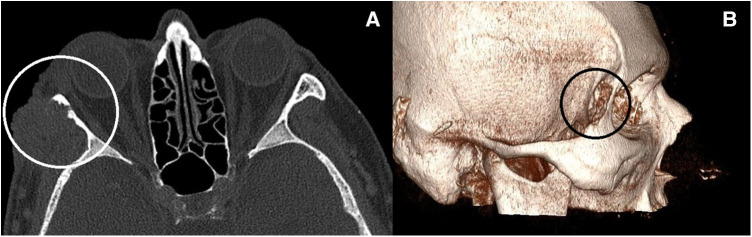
(A,B) Orbital CT scan—tumor eroding the bone.
